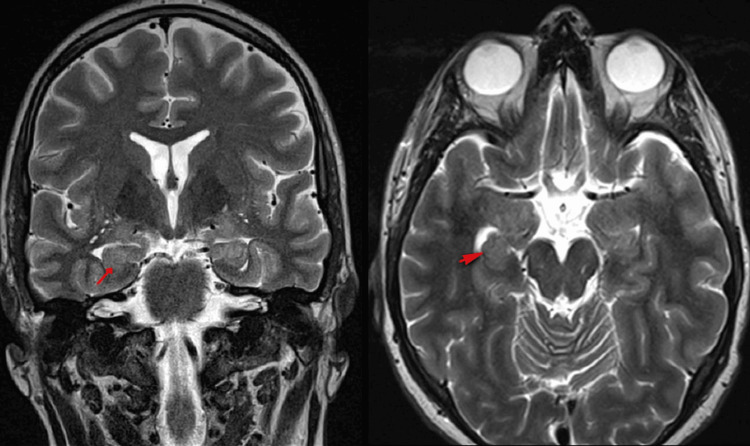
Seizure protocol an MRI with and without contrast was done. The axial and coronal T2-weighted images show a bilateral, rounded-shaped hippocampus with a loss of interdigitations likely related to hippocampal malrotations.
